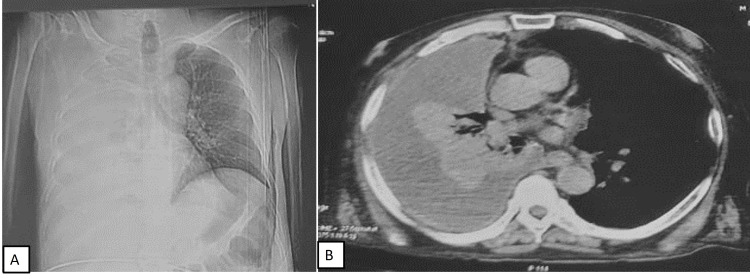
Chest radiograph (A) and chest CT (B) showing right-sided pleural effusion with passive collapse.
